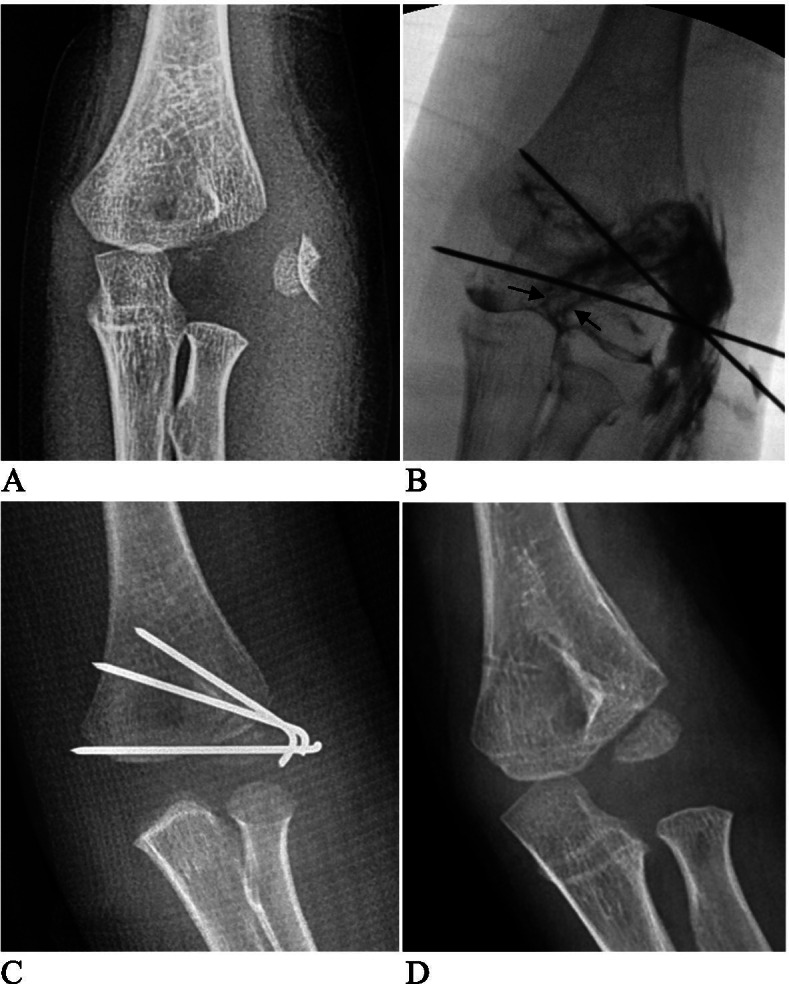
A Song stage 5 and Milch typeILCHF of a 5 years old boy. A Pre-operative anterior-posterior X-ray. B Intro-operative arthrogram after CRPP showed unacceptable fracture gap (>2 mm, black arrows), then ORIF was performed. C X-ray post ORIF. D X-ray 6 months post ORIF after internal pins’ removal showed a nearly normal bone structure
